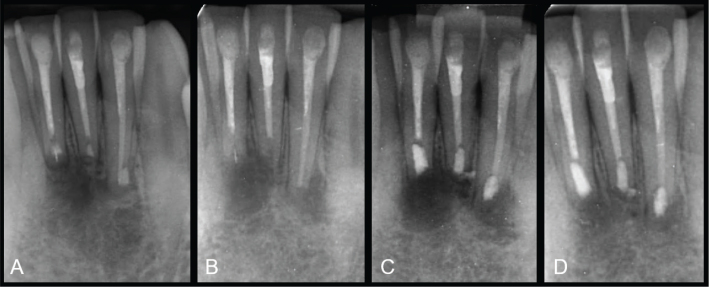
Retrograde fillings with IRM in lower front teeth in 2009 (A), follow-up in 2020 with loss of contrast of the retro-fillings and secondary infection (B), postoperative radiograph with TotalFill BC Putty (FKG, Switzerland) 2021 (C), and follow-up 5 months later with healing (D).
